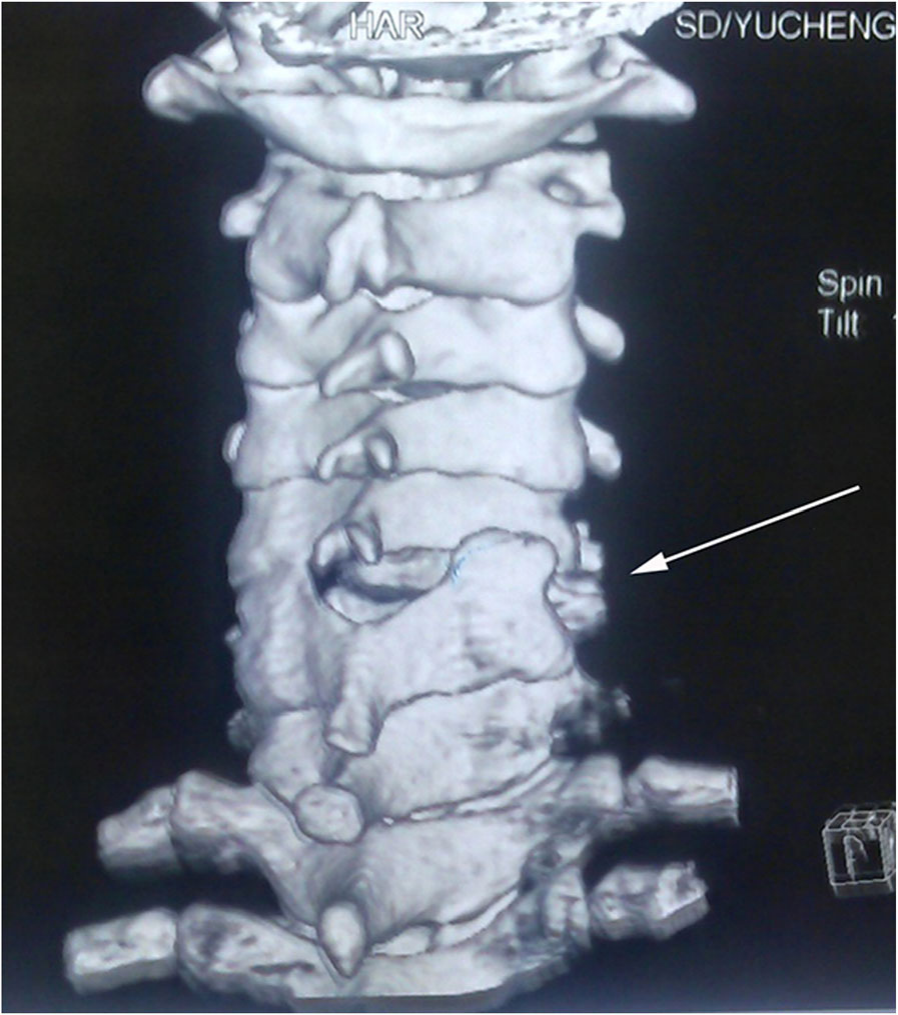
Three-dimensional computed tomography scan before treatment: locked facets of C5/6 (arrow)
